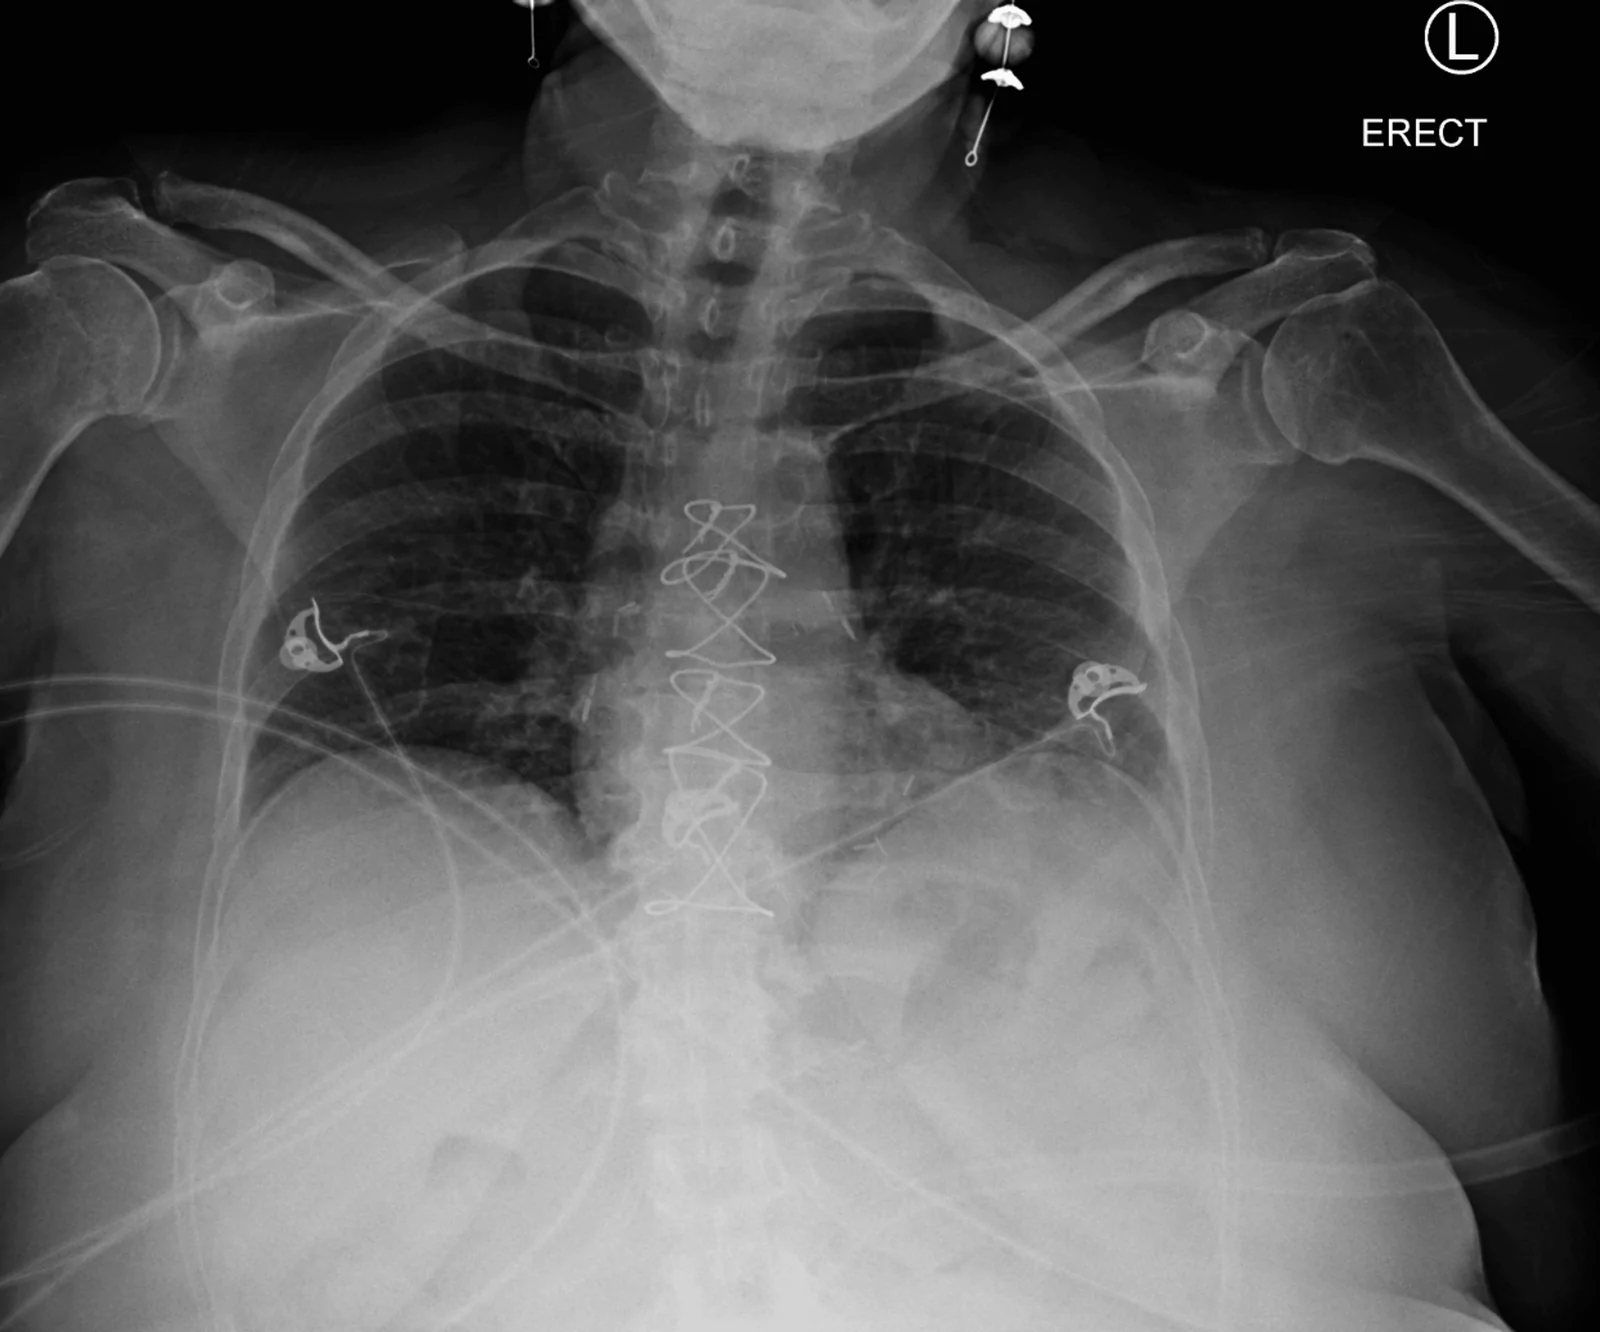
Chest X-ray. Negative chest X-ray showing clear lungs.
